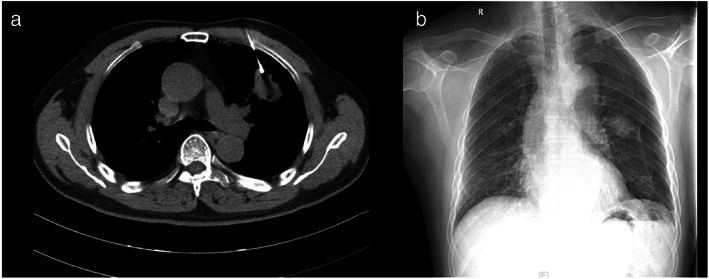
Depicts the case of a patient with severe pneumothorax requiring tube drainage after computed tomography (CT)‐guided lung puncture. A 64‐year‐old patient with lung adenocarcinoma was found to have a nodule in the left upper lobe on CT imaging; the patient presented with 40% lung collapse after lung puncture, and his symptoms were relieved after closed chest drainage
